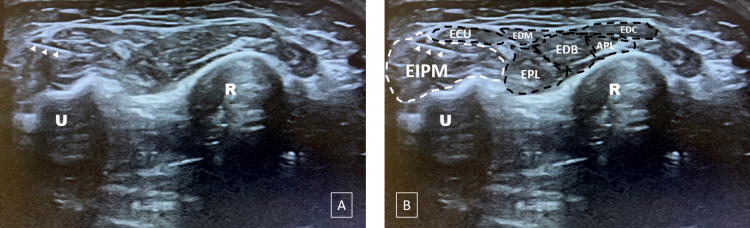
Ultrasound image (A) Ultrasound section in the distal part of the forearm without and (B) with delineation of muscle structures. The extensor indicis proprius muscle (EIPM) is located at the deep medial side of the posterior compartment of the forearm. This ultrasound section shows also the electromyography (EMG) needle (arrowheads) in the EIPM. APL: abductor pollicis longus; ECU: extensor carpi ulnaris; EDB: extensor digitorum brevis; EDC: extensor digitorum communis; EDM: extensor digitorum mini; EIP: extensor indicis proprius; EPL: extensor pollicis longus; R: radius; U: ulna Note: These images were taken from a voluntary medical resident.
